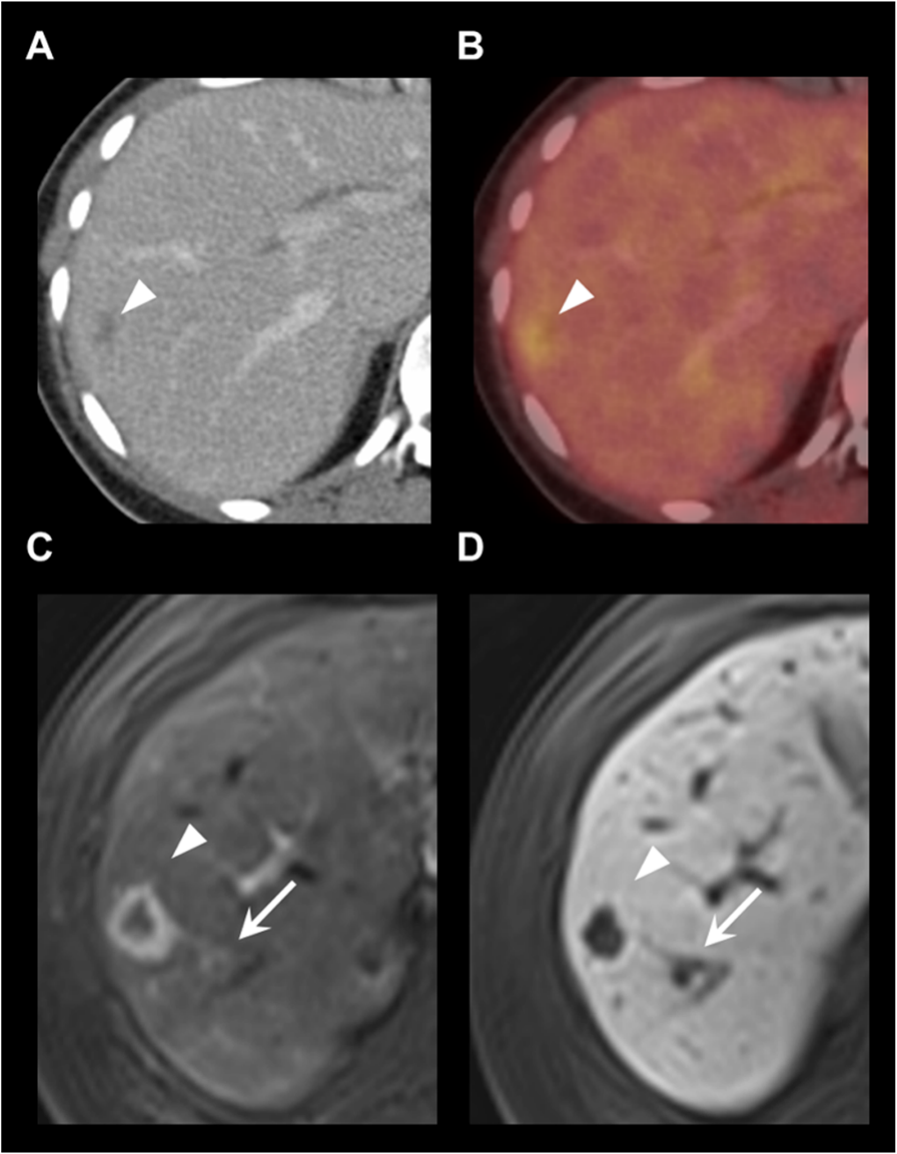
Detection of an additional, ^18^F-DOPA-PET/CT-occult metastasis on gadoxetic acid-enhanced MRI. a Contrast-enhanced CT. b
